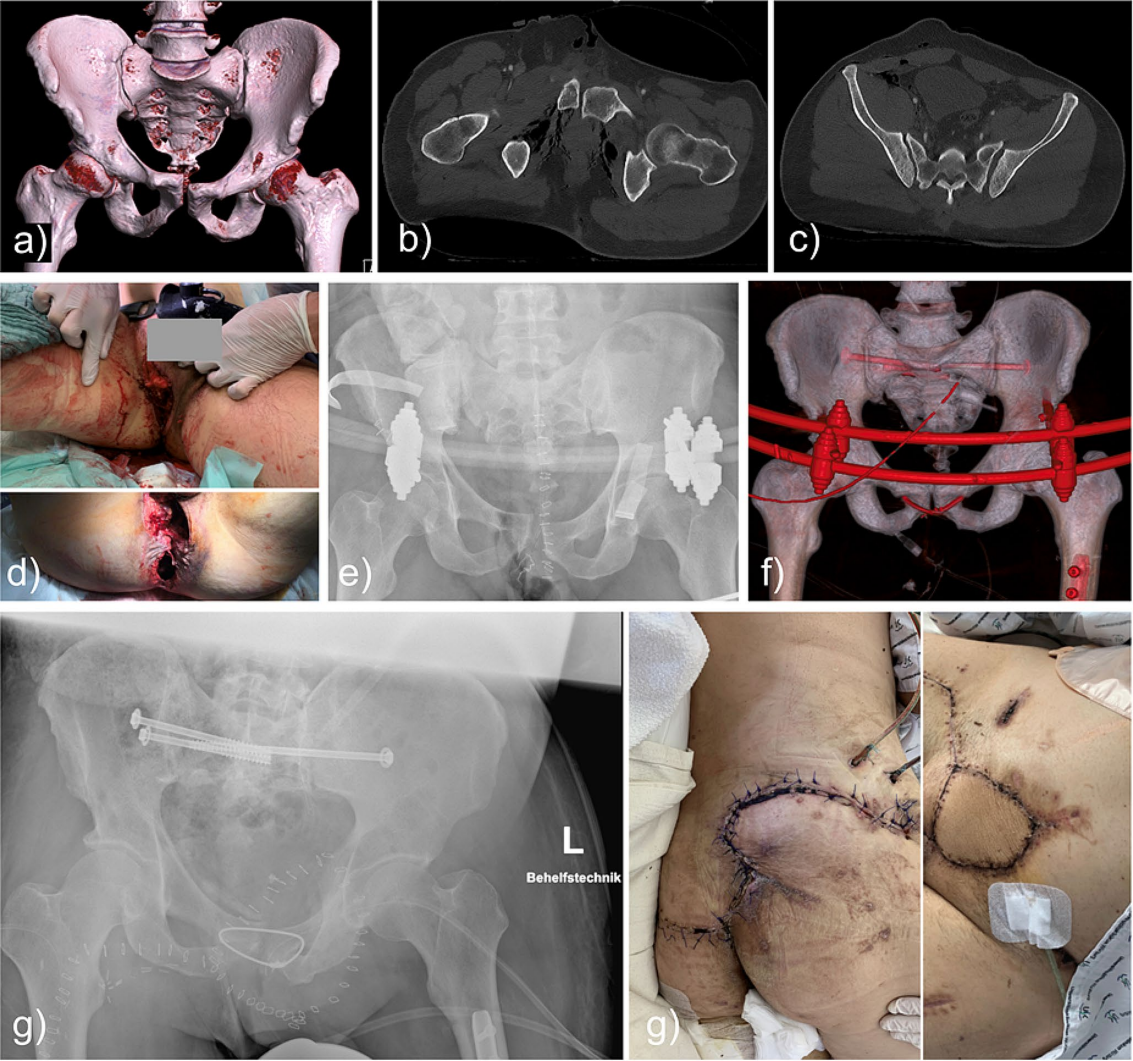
Case#1: a-c) Open pelvic injury with disruption of the pubic symphysis and the iliosacral joints on both sites. Severity of the injury may be underestimated in the CT scan due to the pelvic binder. Air within the true pelvis indicates the open injury. d) Pubic injury with rupture of the rectum, rupture of the pelvic floor and injury to the urethra. The wound was contaminated with wood. Surgical debridement through a midline incision and external fixation followed. e) X-ray of the supraacetabular fixation. f) Subsequent infection required multiple surgical wash outs and vacuum wound therapy. TOCF was chosen instead of a plate in combination with external fixation and SI-screws. g) Removal of the fixator was necessary because of a pin-tract infection. Finally, plastic surgeons were able to close the wound with flaps
